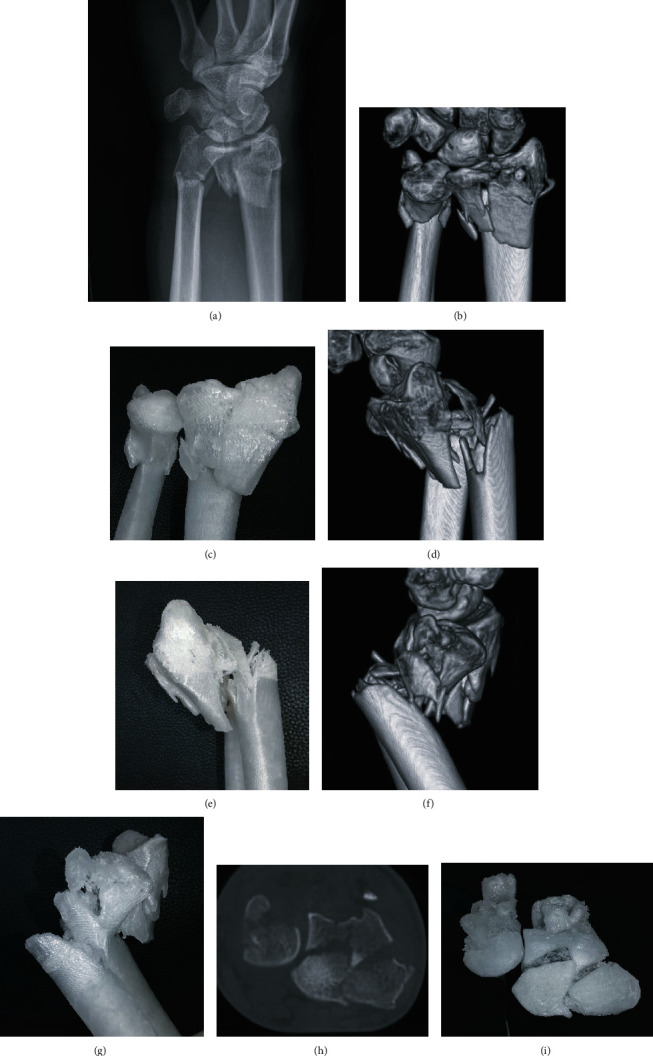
(a) Dorsopalmar radiographic view of a displaced, multifragmentary, intra-articular fracture of the distal radius, and ulna of a 45-year-old female. (b, c) Coronar view of the 3D-CT reconstruction and the equivalent view on the 3D-printed Polypropylene-model. (d, e) Sagittal view (radial aspect) of the 3D-CT reconstruction and the equivalent view on the 3D-printed Polypropylene-model. (f, g) Sagittal view (ulnar aspect) of the 3D-CT reconstruction and the equivalent view on the 3D-printed Polypropylene-model. (h, i) Axial CT-projection and the equivalent view on the 3D-printed Polypropylene-model allowing an overview on the intra-articular key fragments of the distal radius fracture.
